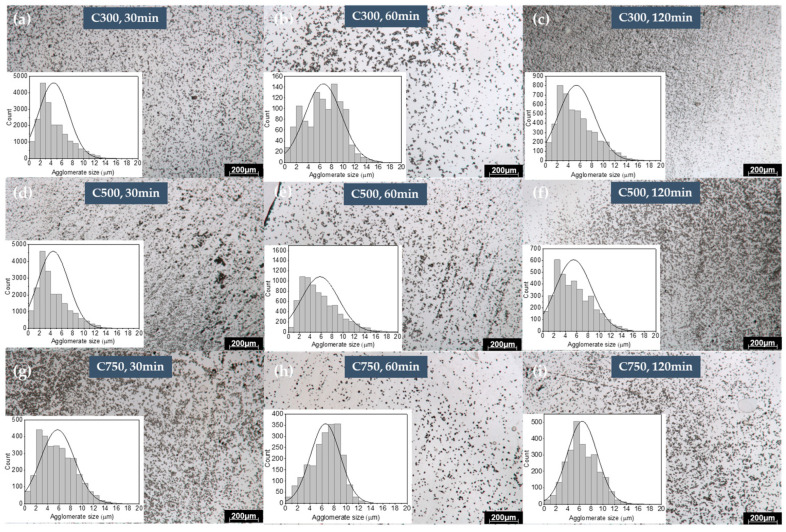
TOM images of samples with 12% loading and variable ultrasonication time (a–c) corresponds to C300 (30, 60, and 120 min), (d–f) corresponds to C500 (30, 60, and 120 min), and (g–i) corresponds to C750 (30, 60, and 120 min). Each image presents the histogram of the agglomerate sizes.
